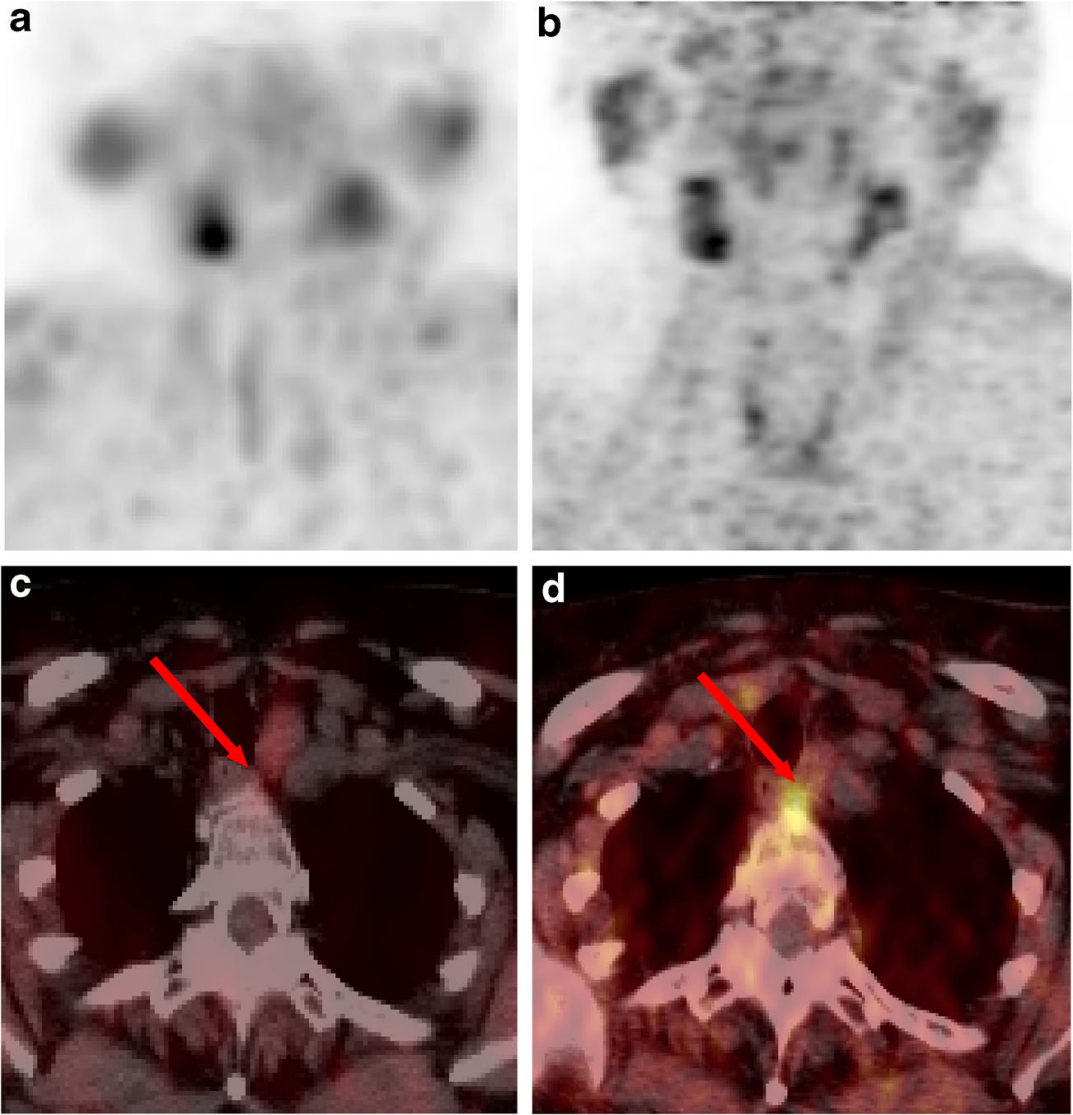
MIBI SPECT/CT and FCH PET/CT images of a 68-y-old female patient with PHPT, an inconclusive cervical ultrasound report (dubious right inferior focus only visible on one plane) and a negative MIBI SPECT/CT. (a) MIBI SPECT maximum-intensity-projection, (c) MIBI SPECT/CT transverse slice, (b) FCH PET maximum-intensity-projection, and (d) FCH PET/CT transverse slice. The MIBI and FCH MIP images (a, b) did not show clear focal uptake in the lower neck. However, the FCH PET/CT transverse slice (d) showed increased FCH uptake in a small ectopic deep-laying left-sided PTA in the upper mediastinum, which was negative on MIBI SPECT/CT (c) (red arrows). Successful ectopic parathyroidectomy was performed, leading to cure
